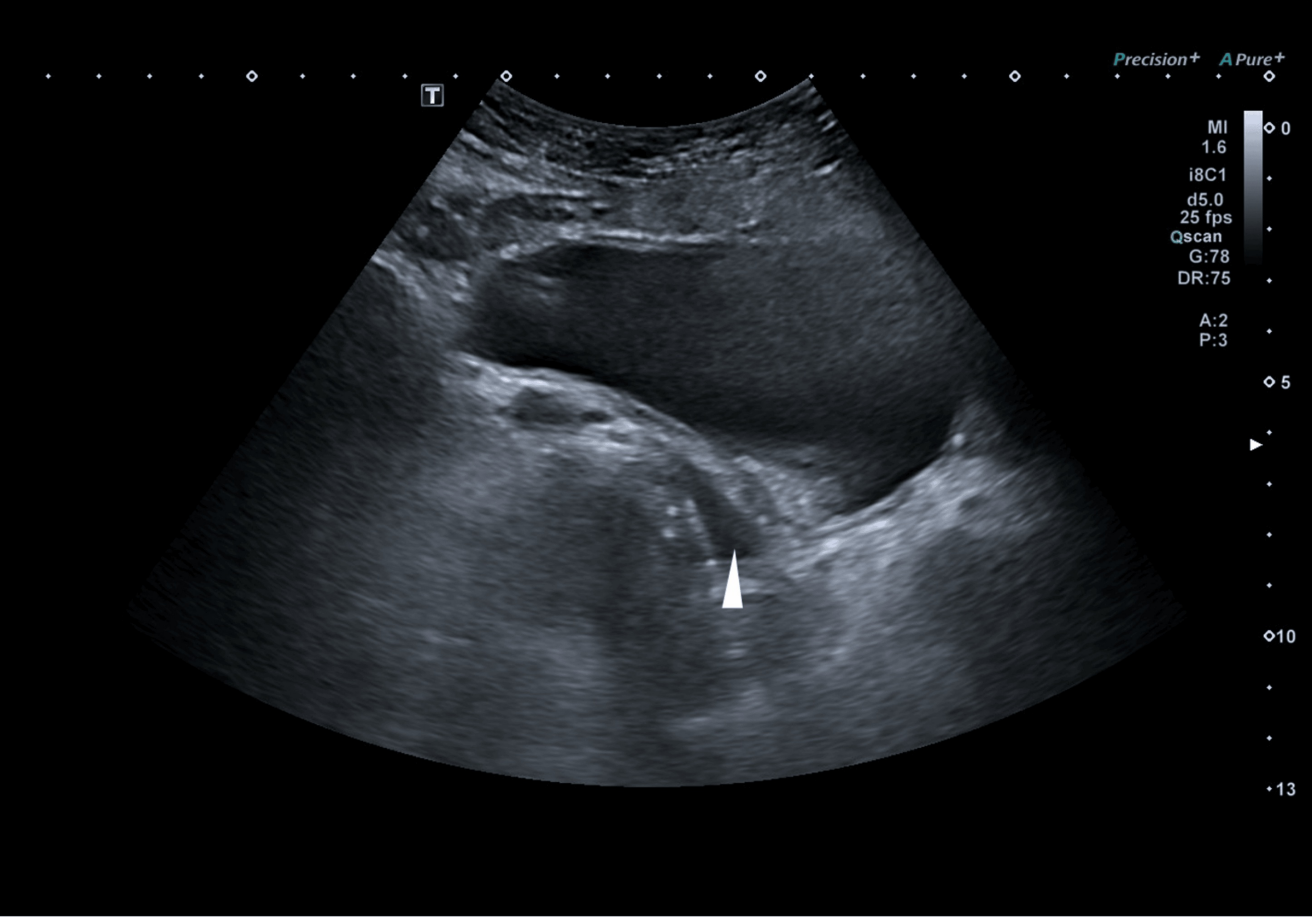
The abdominal ultrasound shows fluid collection, predominantly an anechoic portion of the large image occupying the abdomen, and a well-defined interface between the superior anechoic fluid and the denser, echogenic content (white arrow).
